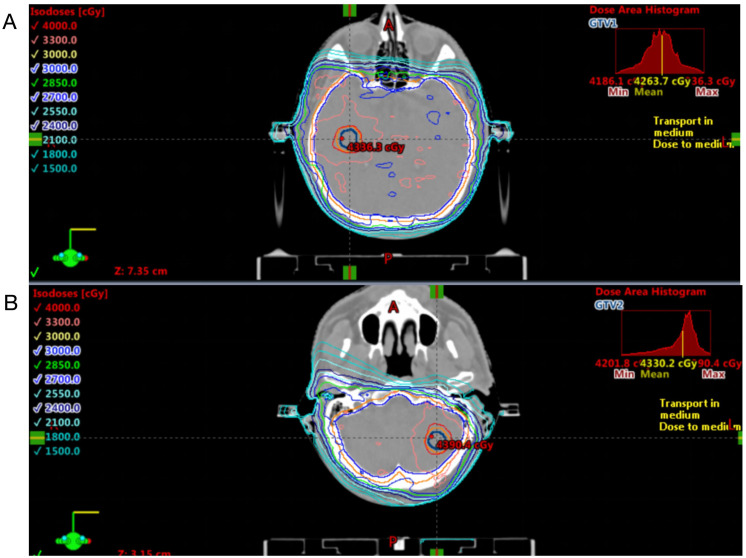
Radiotherapy dose distribution of GTV1 (A) and GTV2 (B) visualized on planning CT.
